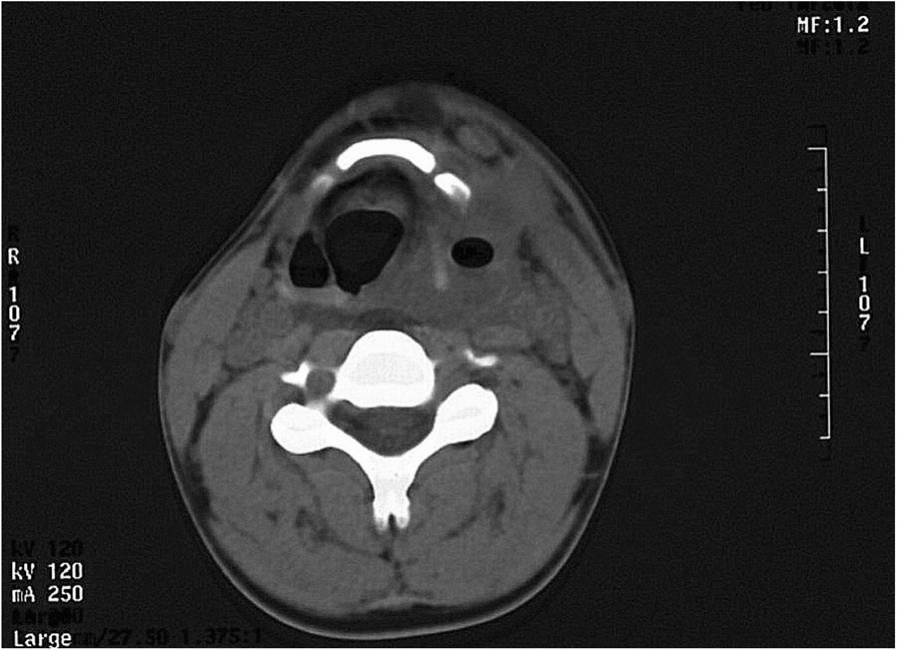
A CT image. Air pocket within the swelling tissues of the *left* neck
